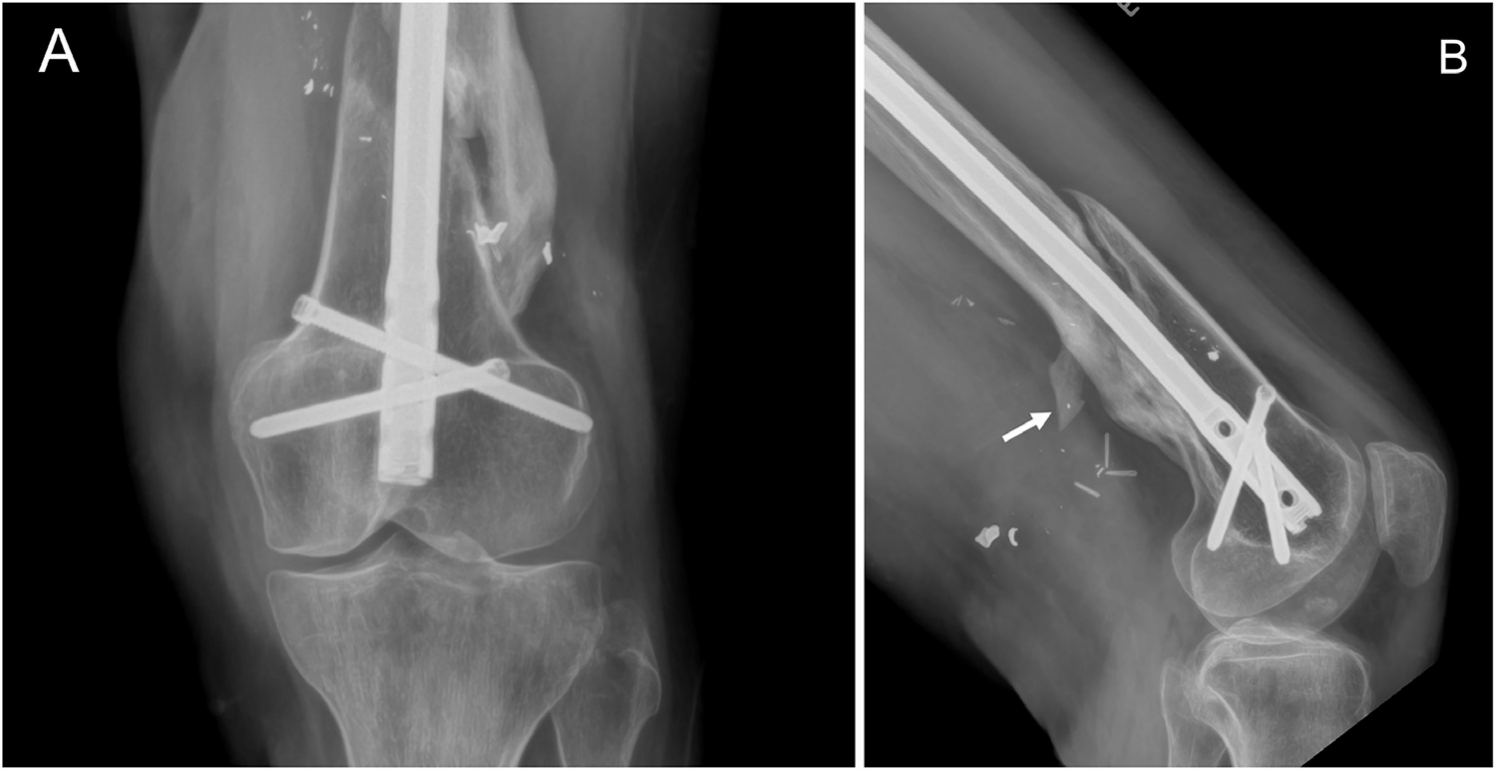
AP (A) and lateral (B) radiographs of the distal left femur 10 months after injury demonstrate improved fracture alignment and healing after placement of an intramedullary nail. The posteriorly directed fracture fragment remains unchanged in position after fixation (arrow) with surrounding metallic debris.
